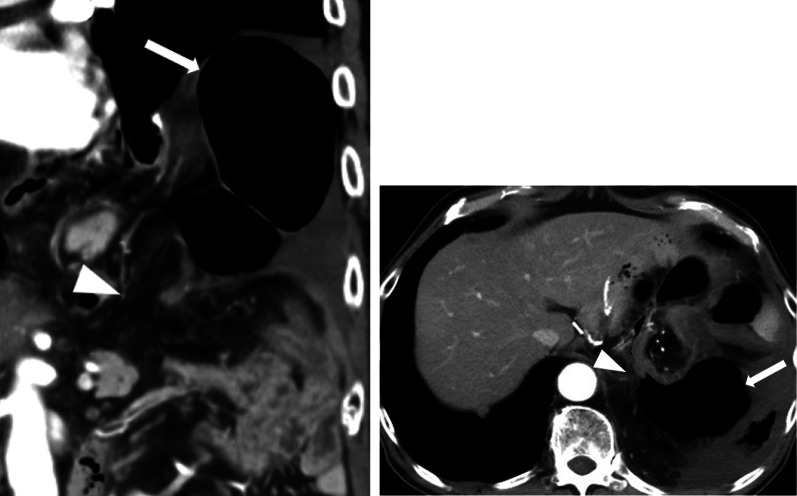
Computed tomography with intravenous contrast demonstrating a transverse colon (arrow) that has herniated into the left hemithorax through the hernial defect (arrowhead)
